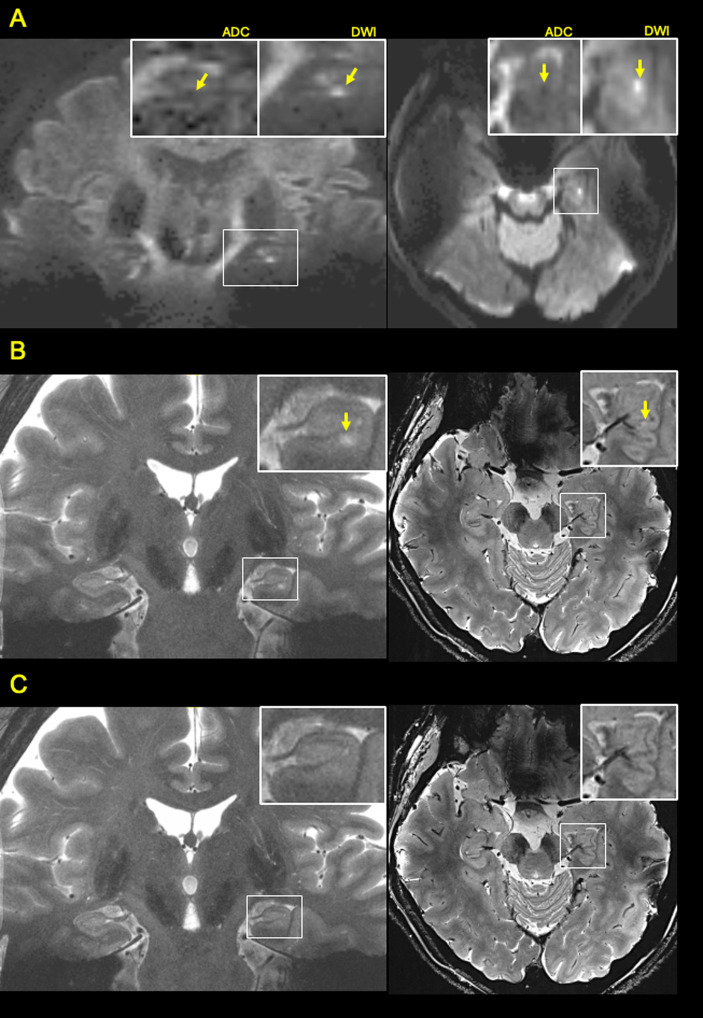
Serial 7 T MRI findings in Case 2 (delayed acute acquisition). (A) Acute 7 T diffusion-weighted imaging (DWI) obtained 102 h after symptom onset demonstrates a focal CA1 hyperintensity (arrow). Corresponding apparent diffusion coefficient (ADC) maps show focal hypointensity at the site of the DWI lesion, confirming restricted diffusion. Magnified views of the lesion are shown in the upper-right corner of each image; the left inset displays the ADC map, and the right inset displays the DWI image. (B) Concurrent 7 T T2-weighted imaging shows a corresponding cortical-layer hyperintensity within the hippocampal CA1 subfield (arrow), visible on both coronal and axial views despite the delayed acquisition. (C) Follow-up 7 T T2-weighted imaging performed on day 14 shows complete interval resolution of the CA1 signal abnormality.
